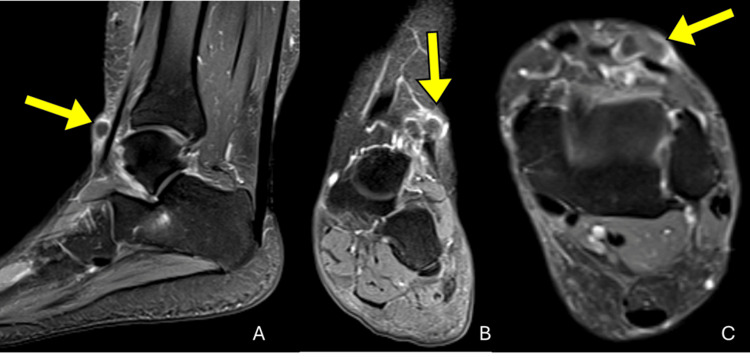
Post-contrast T1-weighted MRI (fat-saturated) of the left ankle showing a pericapsular ganglion cyst (yellow arrows). (A) Sagittal, (B) coronal, and (C) axial planes demonstrate a well-defined, multilobulated lesion with peripheral enhancement, consistent with a ganglion cyst. The lesion is located in the subcutaneous tissues overlying the anterolateral ankle, adjacent to the deep peroneal nerve.
